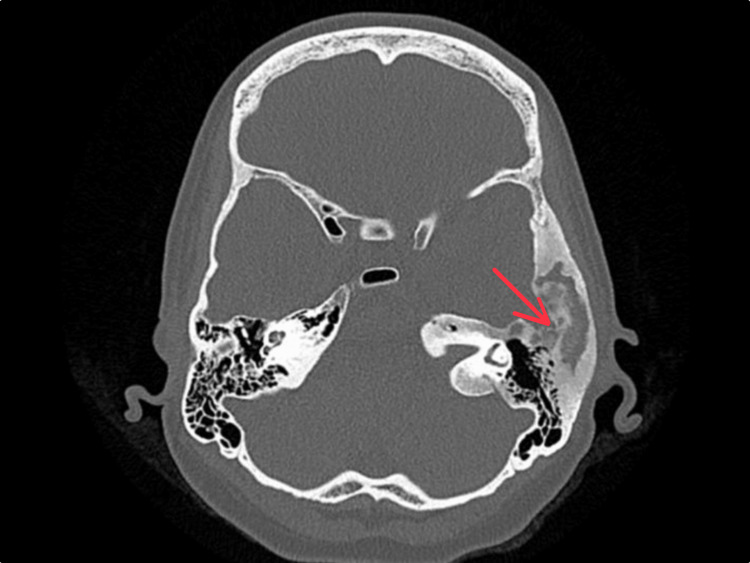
CT Temporal Bones, Axial View Axial CT of the temporal bone demonstrating the characteristic ground-glass appearance of fibrous dysplasia in the left temporal bone (red arrow).
